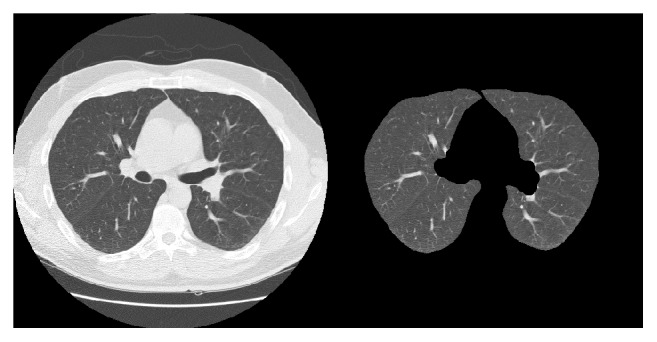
Original CT image and the result after applying the preprocessing stage to isolate the lungs.
